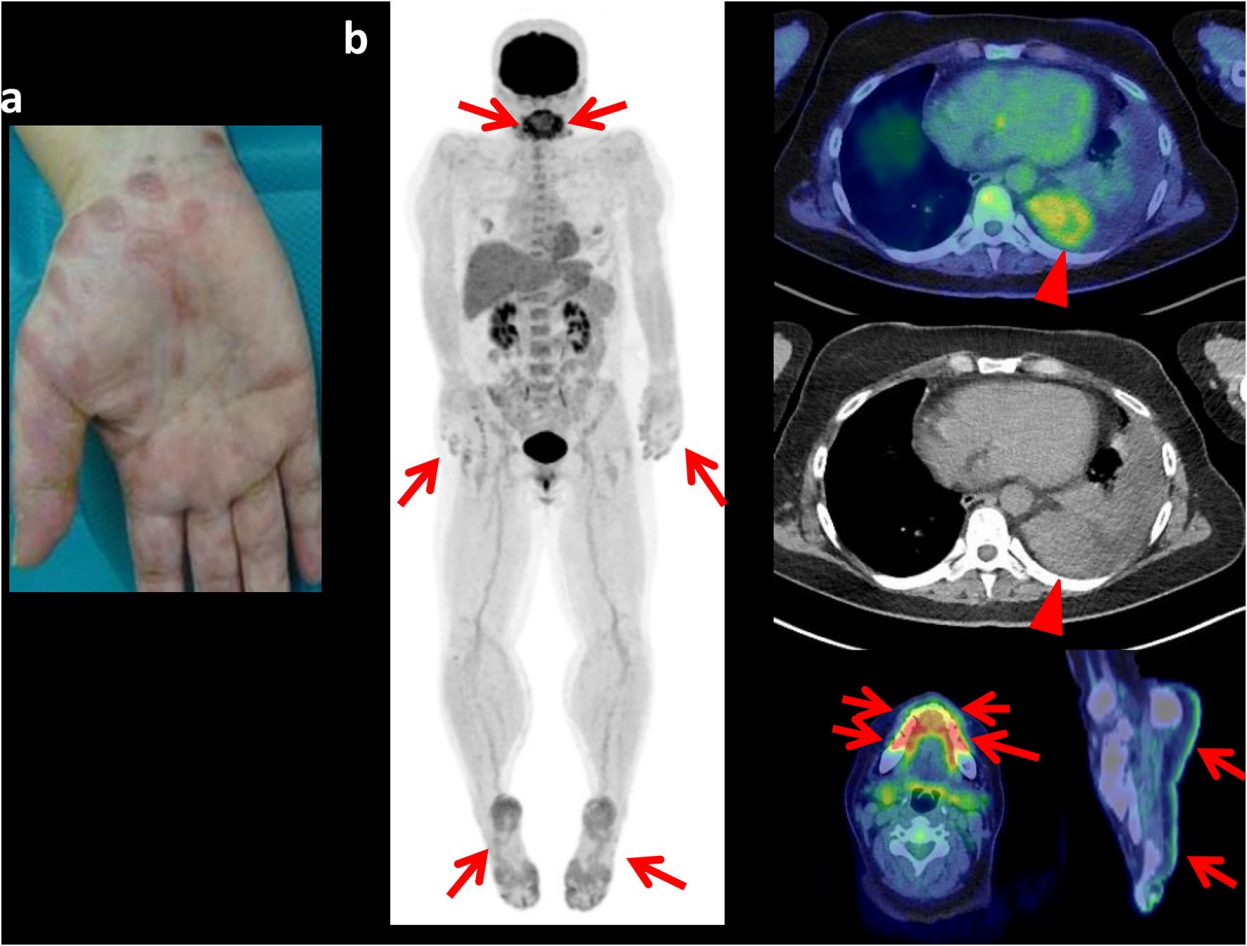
Cutaneous manifestation of paraneoplastic pemphigus at the hand in a 45 year-old female who presented with oral mucosal and lip lesions and polymorphous cutaneous eruption including the both hands and feet (a). Whole-body FDG PET maximum projection image showed abnormal FDG uptake (SUVmax 7.3) in the mouth/lips lesions, also abnormal uptakes (SUVmax6.9) in the both hands and feet (b; arrows). Cross-sectional FDG PET/CT images showed FDG-avid (SUVmax 4.0) mass in the left thorax (arrowhead) and abnormal FDG uptakes in the lip/mouth lesions and cutaneous lesion of the feet (arrows). Later, this patient had surgical resection of the thoracic FDG-avid mass which was diagnosed as hyaline vascular-type Castleman disease
